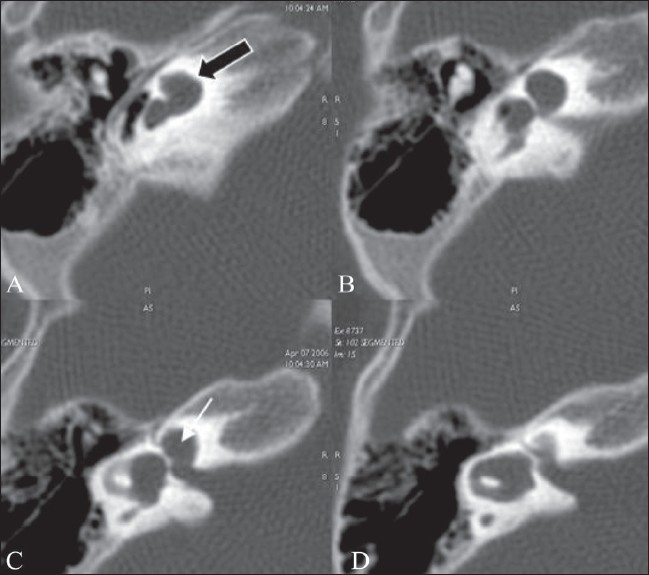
Incomplete partition type I. Successive axial HRCT scans show findings of an incomplete partition type I deformity, with a cystic appearance of the cochlea, lacking the entire modiolus (block black arrow in A) and a cystic vestibule (white arrow in C)
